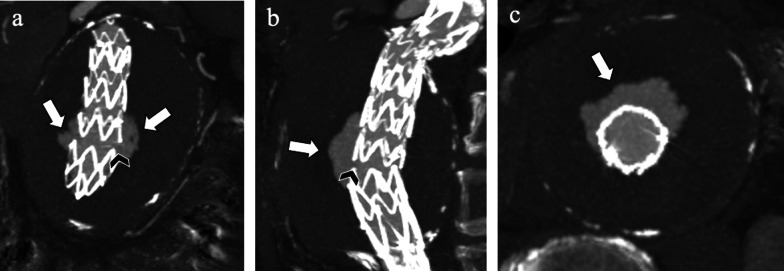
85-year-old man with a probable rupture of PTFE (polytetrafluoroethylene) graft material and *type IIIa* endoleak. a Coronal, (b) sagittal, and (c) axial maximum intensity projection CT angiography images show contrast agent outside stent-graft (arrows) consisting in type III endoleak caused by probable PTFE fabric erosion (arrowheads)
